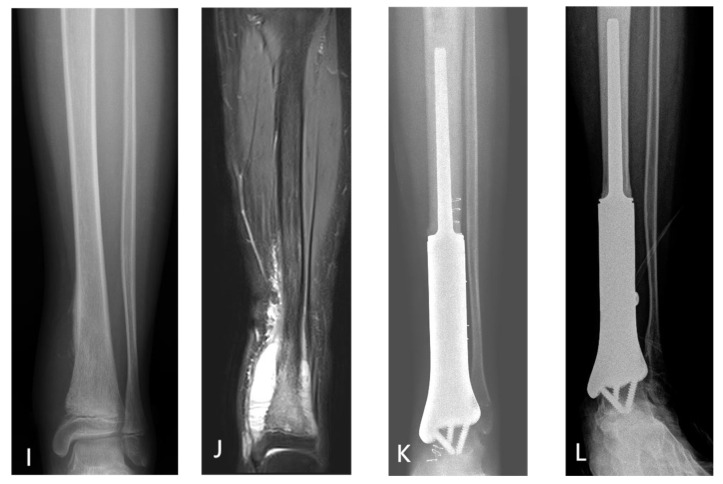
(A–D) A 15-year-old female with osteosarcoma in the right femur underwent biological reconstruction using liquid nitrogen inactivated replantation combined with an allogeneic fibular graft. (A,B) X-ray and MRI showed the tumor involvement in the right femoral. (C) Immediate postoperative X-ray image. (D) Five-year postoperative X-ray image. (E–H) A 17-year-old male with osteosarcoma in the right tibia underwent biological reconstruction using liquid nitrogen inactivated replantation combined with autologous fibular graft. (E,F) X-ray and MRI showed the tumor involvement in the right tibia. (G) Immediate postoperative X-ray image. (H) Five-year postoperative X-ray image. (I–L) A 12-year-old male diagnosed with osteosarcoma in the left tibia underwent reconstruction using a 3D-printed prosthetic implant.
